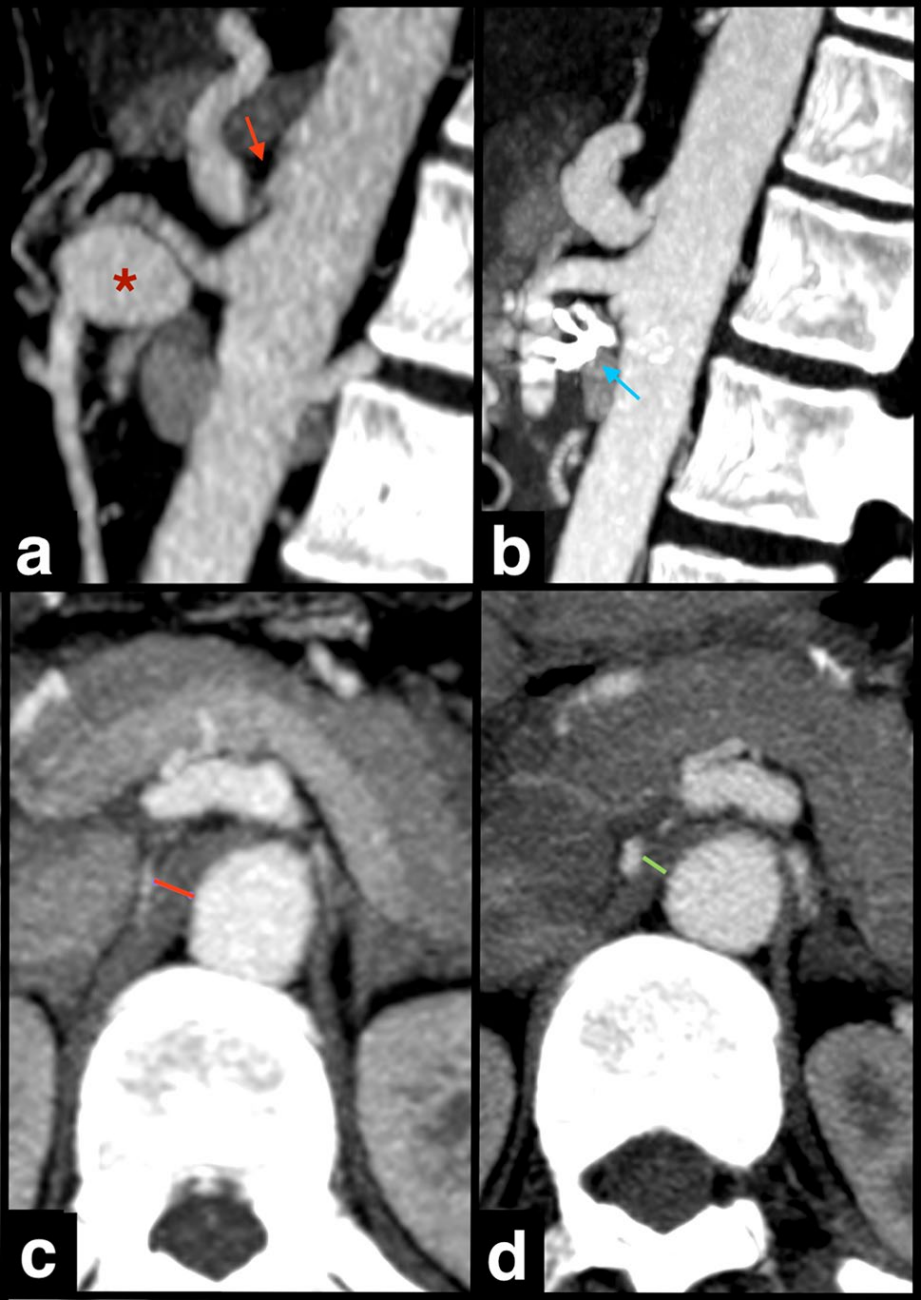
Residual stenosis after surgical incision of the median arcuate ligament (MAL). CTA images from the same patient in Figure, before (a, c) and 3 months after (b, d) surgical MAL incision, performed 16 months after the endovascular treatment of the pancreaticoduodenal artery aneurysm (*). Sagittal MIP CTA images (a, b) show the hook-shaped appearance at the origin of the celiac artery (arrow in A), and the coils within the embolized PDA aneurysm (*). Axial CTA images (c, d) show reduction of the maximum thickness of MAL, measured in the axial plane, at the level of the CA origin (red and green lines). CTA, computed tomography angiography; MIP, maximum intensity projection
